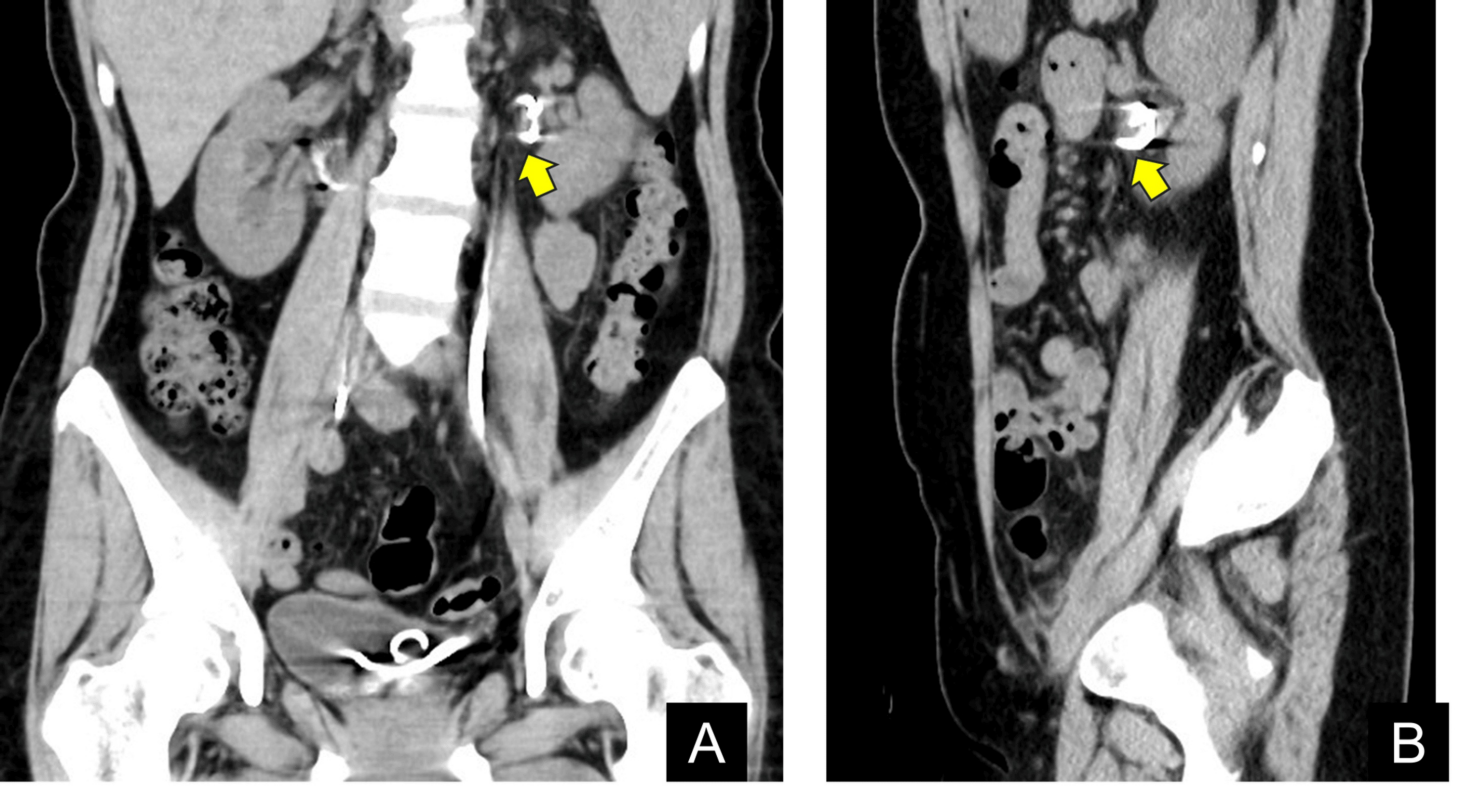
Non-contrast computed tomography (CT) findings. Because of a contrast media allergy, only a non-contrast CT was obtained; the images suggest possible embedding of the proximal loop of the metallic stent within the renal pelvic mucosa (arrows). A: Coronal image. B: Sagittal image.
